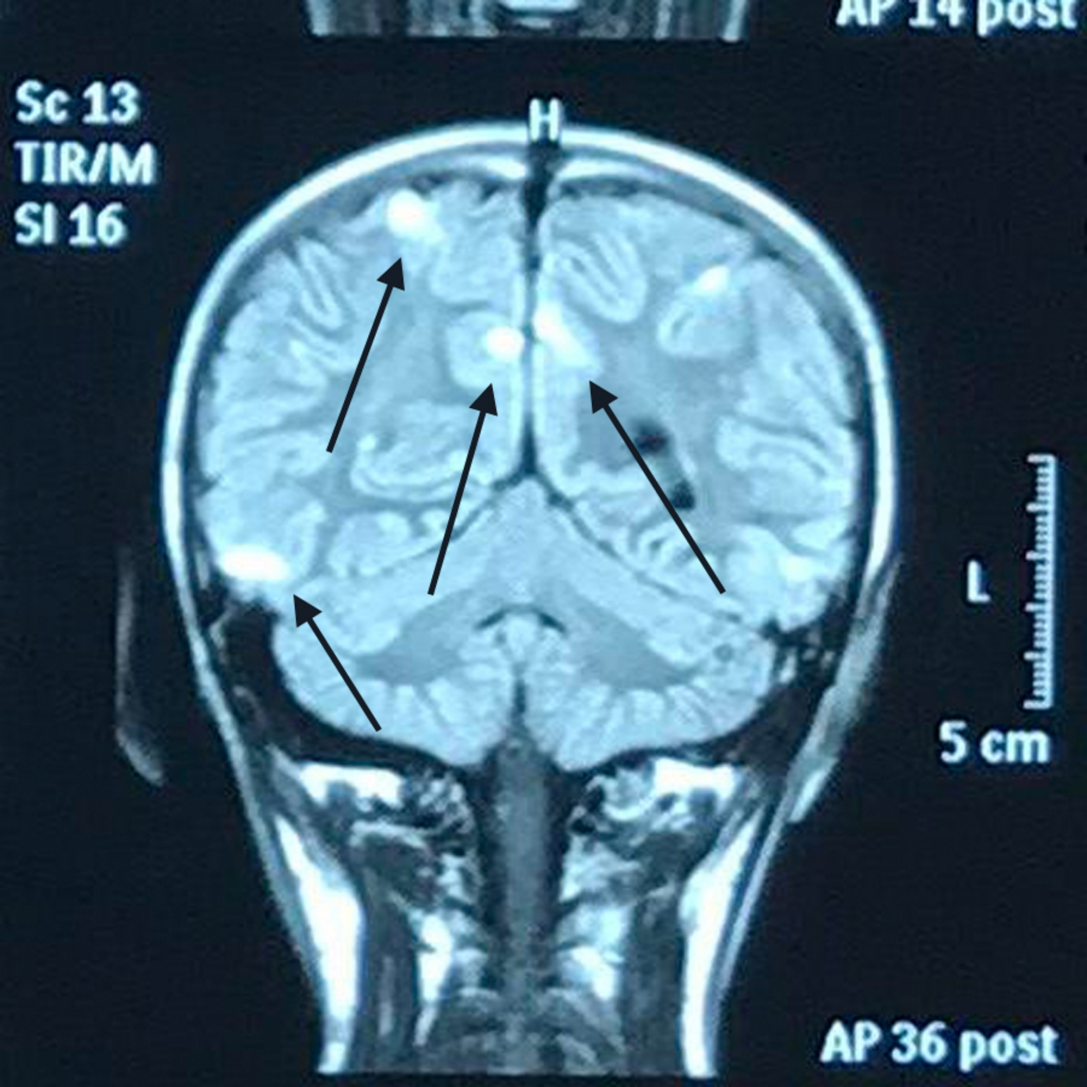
Follow-up magnetic resonance imaging (MRI) of the brain showing abnormal signal intensity areas in the parietal region appearing hyper-intense on fluid attenuation inversion recovery (FLAIR) image.
